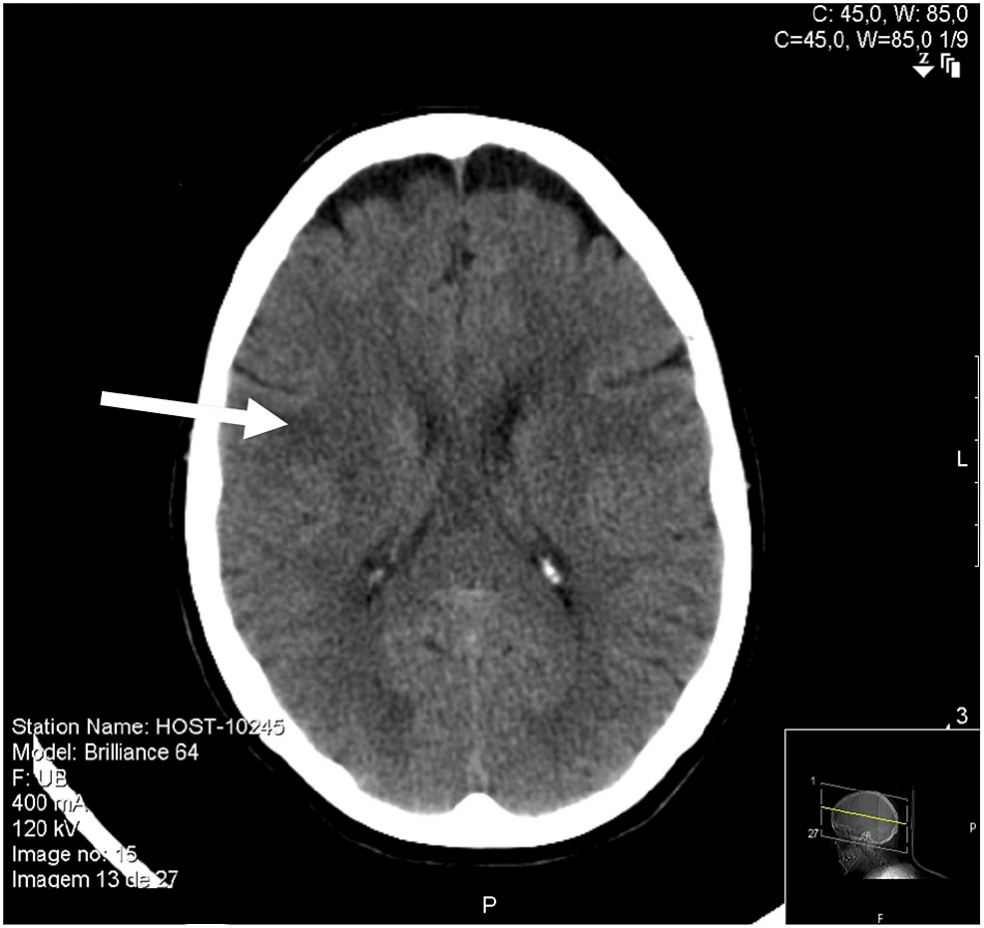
Brain computed tomography performed in the emergency department reveals right frontal cortico-subcortical hypodensity (arrow).
